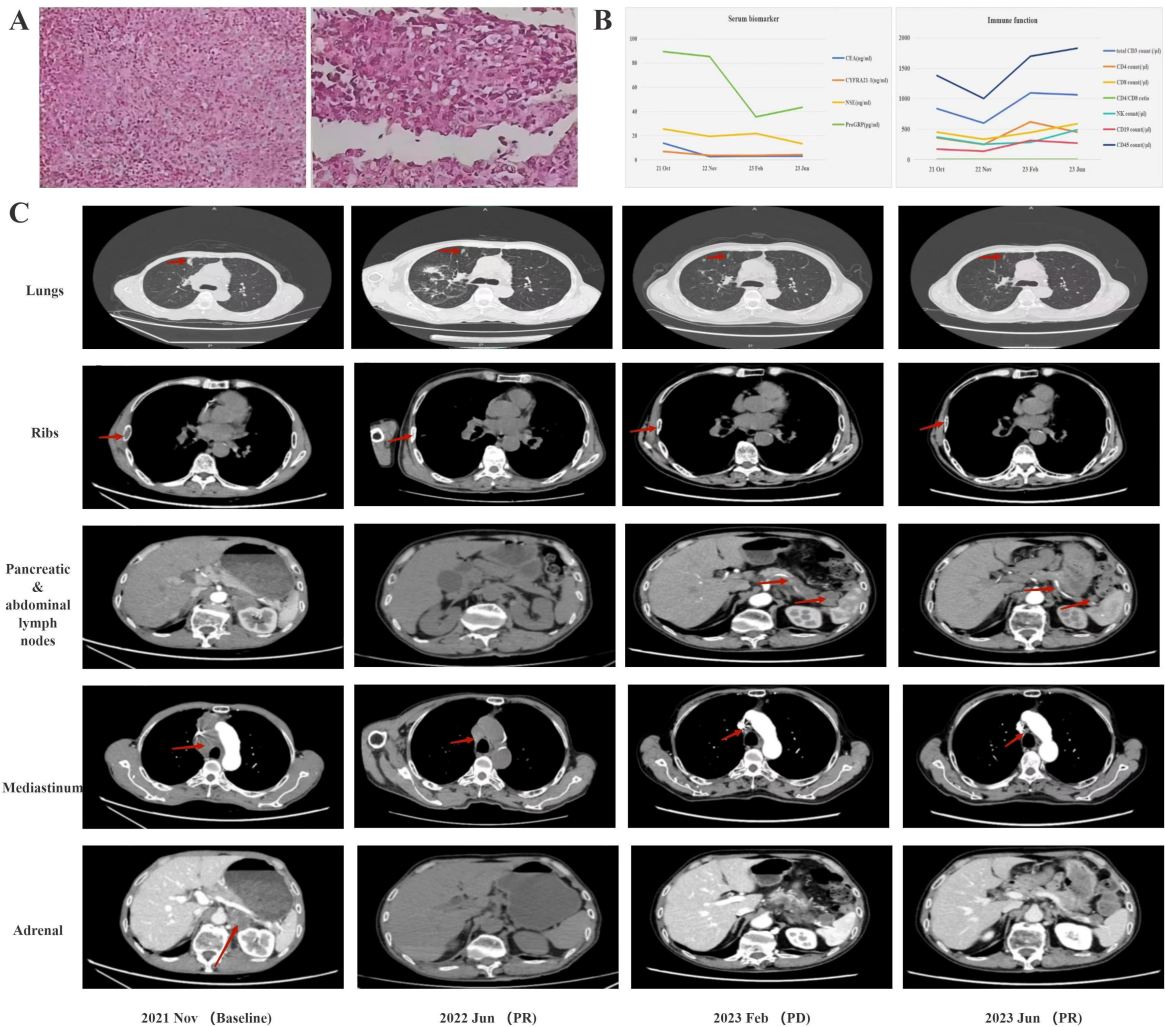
(A) Pathological findings at diagnosis. (B) serum biomarkers during treatment. (C) CT findings during treatment computed tomography.
